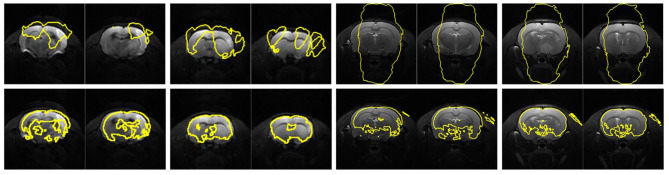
Visual skull stripping results using the original MU-Net (top row) and MedicDeepLabv3+ (bottom row) without retraining. Left columns: DWI Subjects 10 and 21. Right columns: T2WI Subjects 9 and 33
